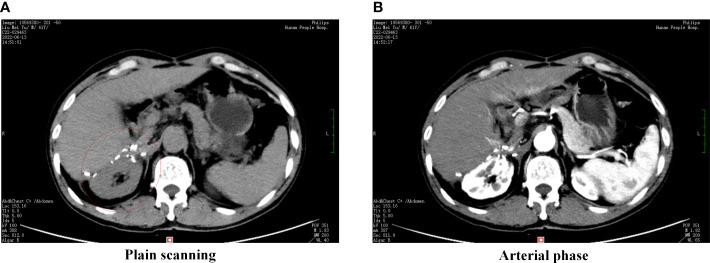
Postoperative abdominal CT. (A) CT scan in plain scanning, (B) CT scan in arterial phase. Postoperative abdominal CT images showed no new mass in the liver-kidney space (red circle).
